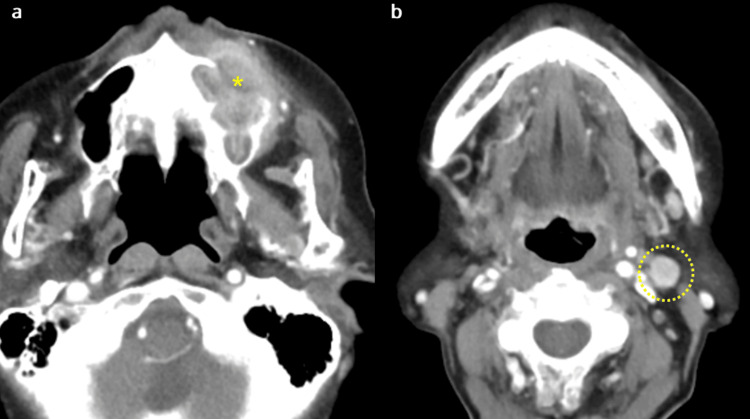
Computed tomography scan a: CT image of the left maxilla showing a lesion with a slightly indistinct margin and heterogeneous internal contrast enhancement. Marked bone resorption of the surrounding maxillary bone resulted in loss of the maxillary sinus floor (yellow asterisk). Increased density within the maxillary sinus is also observed. b: CT image showing enlargement of lymph nodes with contrast enhancement in the left submandibular lymph node.
